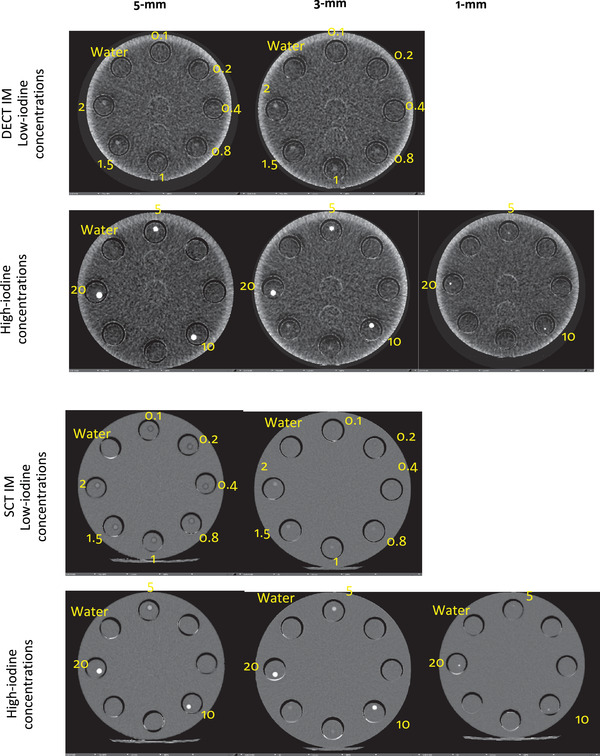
Dual‐energy computed tomography (DECT) and subtraction computed tomography (SCT) images of iodine map (IM) in variety of tube diameters (5, 3, and 1 mm) and iodine concentrations in mg/ml (overlaid in yellow text on the images)
